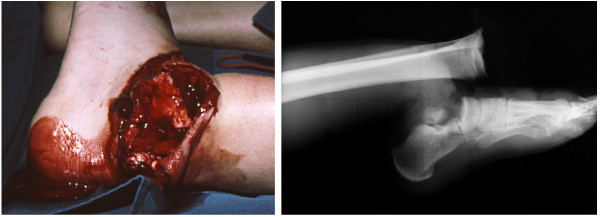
a,b Gustilo IIIc high-energy injury of the ankle and foot with lost talus on admission. Preoperative lateral radiograph of the left ankle and foot on admission (a - left) and skeletal disorders and lost talus (b - right).
